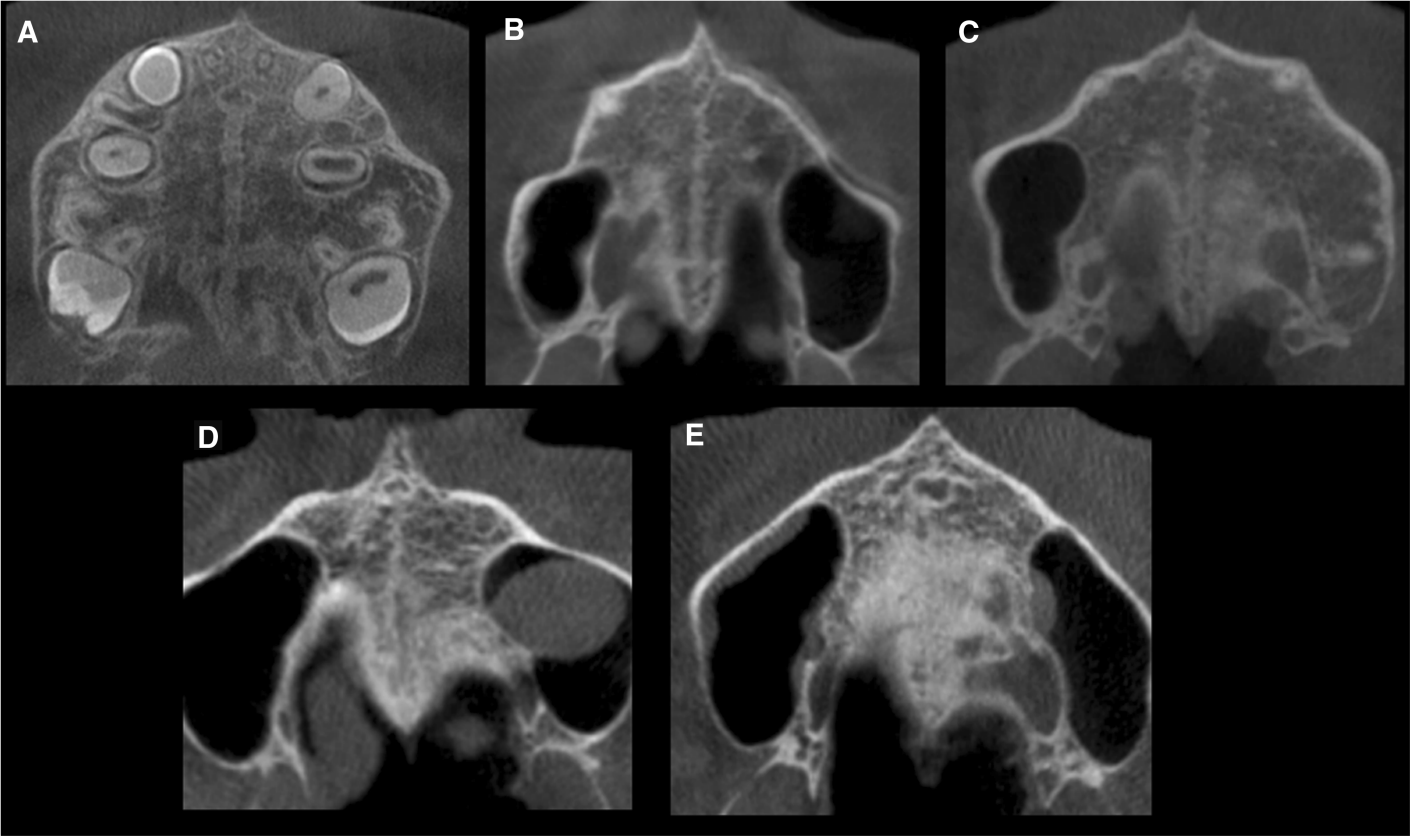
Method of Angieleri et al. [15] in CBCT. a The midpalatal suture is seen as a relatively straight radiopaque line. b The midpalatal suture appears as a scalloped line of high density. c Two radiopaque, scalloped, and parallel lines are separated by areas of low radiographic density. d The palatine bones become more radiopaque, and the suture is not visualized in this sector only is visualized as two scalloped high-density lines at the midline on the palate bone. e It is no longer possible to see the suture along the maxillary and palatine bones, indicating fusion l; fusion has occurred in the maxilla
